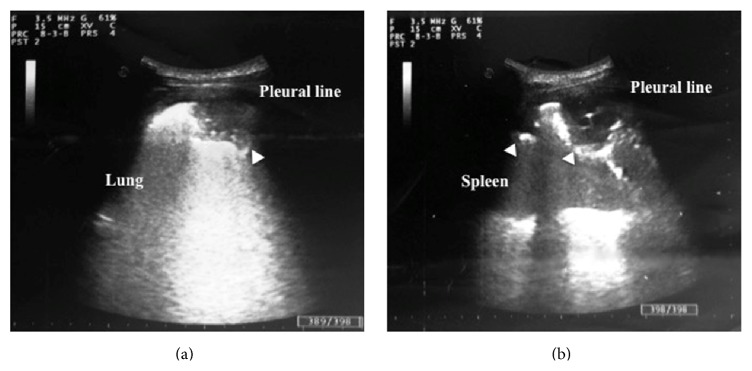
Chest sonography (3.5 MHz convex scanner): hypoechoic, wedge, and rounded shaped, subpleural consolidations (arrowheads) in the right (a) and left lung (b).
